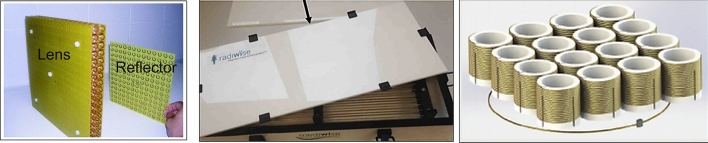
Photographs of various metamaterial-based prototypes for MRI. (Left) A metamaterial lens based on split rings [61], (centre) a structure based on conductive rods within a high permittivity liquid [67], and (right) the first metamaterial MRI structure published based on “swiss rolls” [65]
